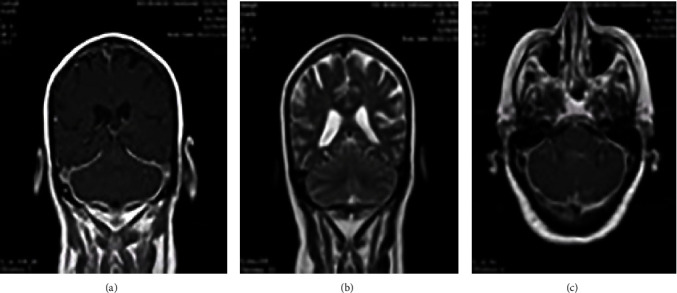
Diffuse smooth thickening and enhancement of the tentorium and adjacent basal dura were observed. Additionally, there was diffuse increased leptomeningeal enhancement in both supra and infra-tentorial parts of the brain, brain MRI, T1 sequence, and coronal view with GAD (a), T2 sequence and coronal view with GAD (b), T1 sequence and axial view with GAD (c).
